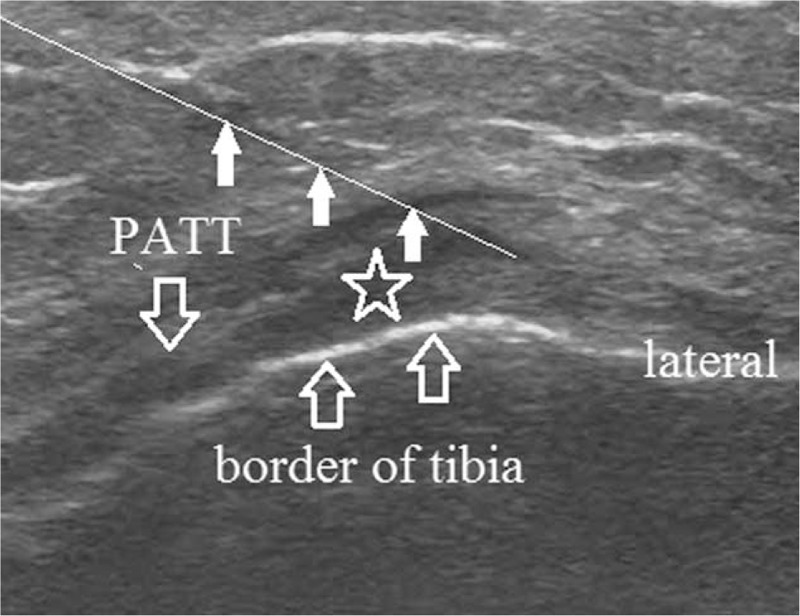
Ultrasound image during injection of polydeoxyribonucleotide in the pes anserine bursa (asterix). White arrow indicates the block needle. PATT = pes anserine tendon thickening.
